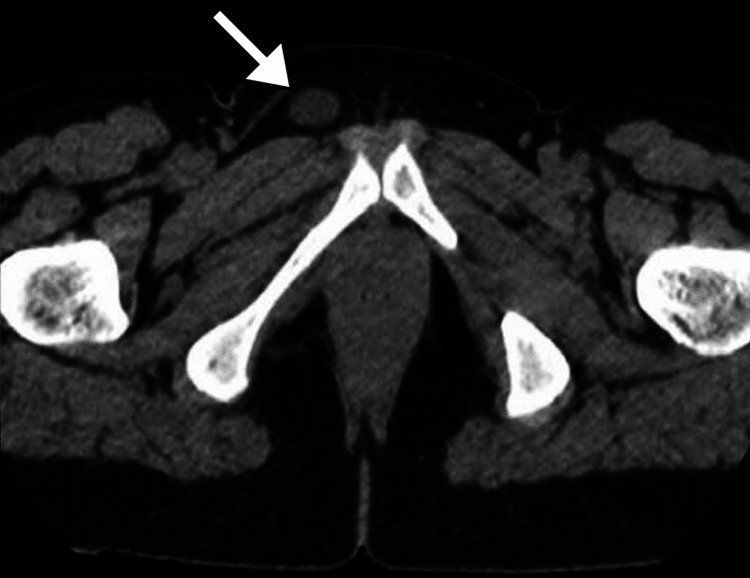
CT image of the pelvis Selected axial images from the CT pelvis scan demonstrated a well-defined, fluid-filled lesion (arrows) in the right inguinal canal, with no evidence of calcification or soft tissue components. CT: computed tomography
